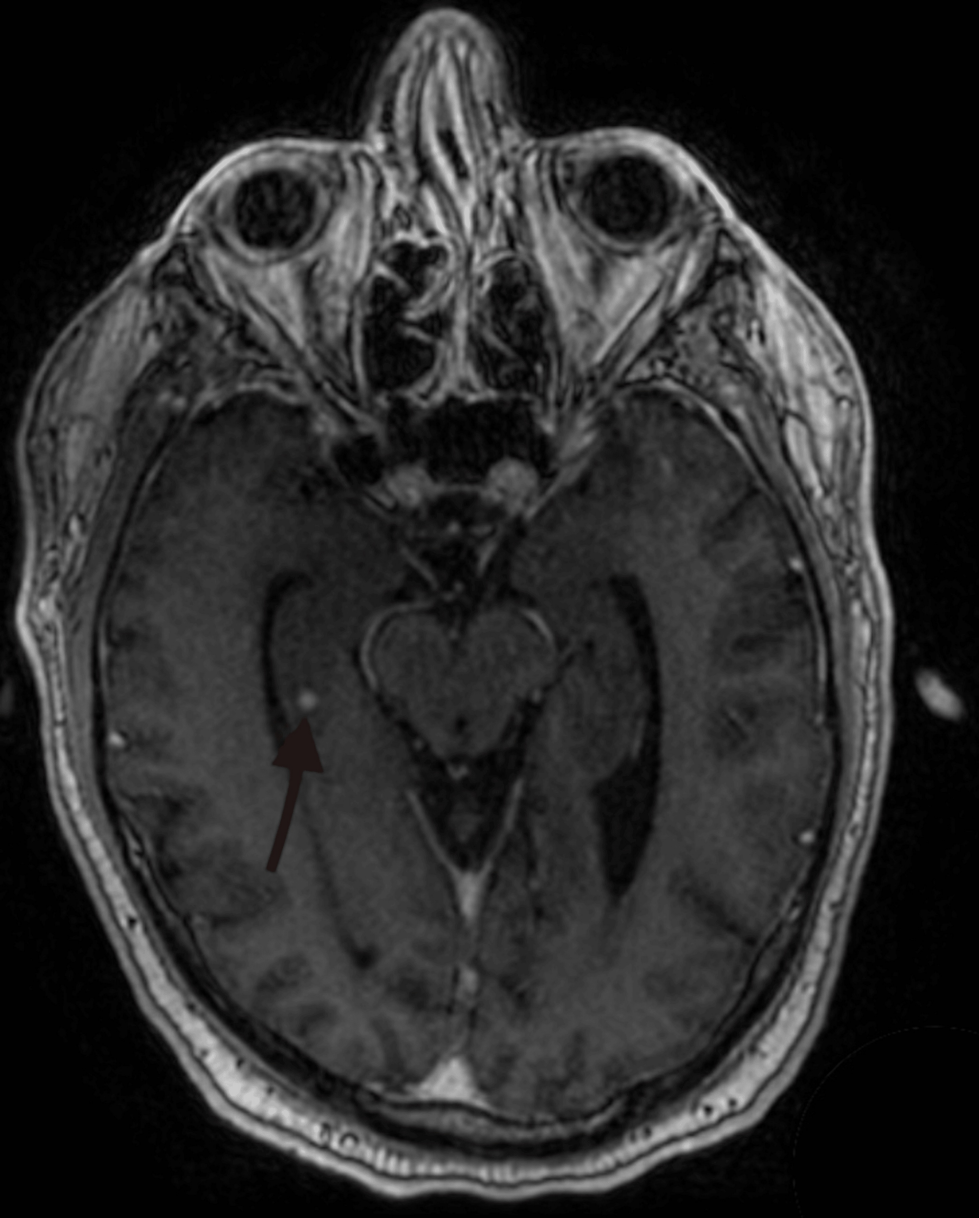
MRI image displaying metastatic lesion in the brain The red arrow depicts a lesion found within the right temporal cortex. MRI: Magnetic resonance imaging
